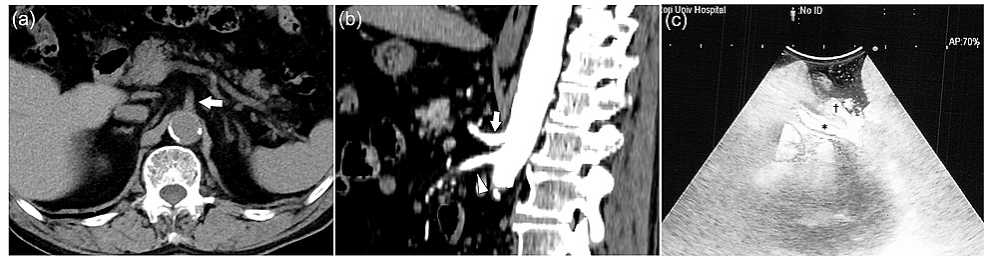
(a) Preoperative plain CT showing no arteriosclerosis around the celiac artery (CA) trunk (arrow). (b) Preoperative contrast-enhanced CT showing no evidence of median arcuate ligament syndrome (MALS). (sagittal view; arrow shows the CA; arrowhead shows the superior mesenteric artery) (c) Intraoperative ultrasonography showing good hepatic arterial blood flow. (* right hepatic artery, † left hepatic artery)
